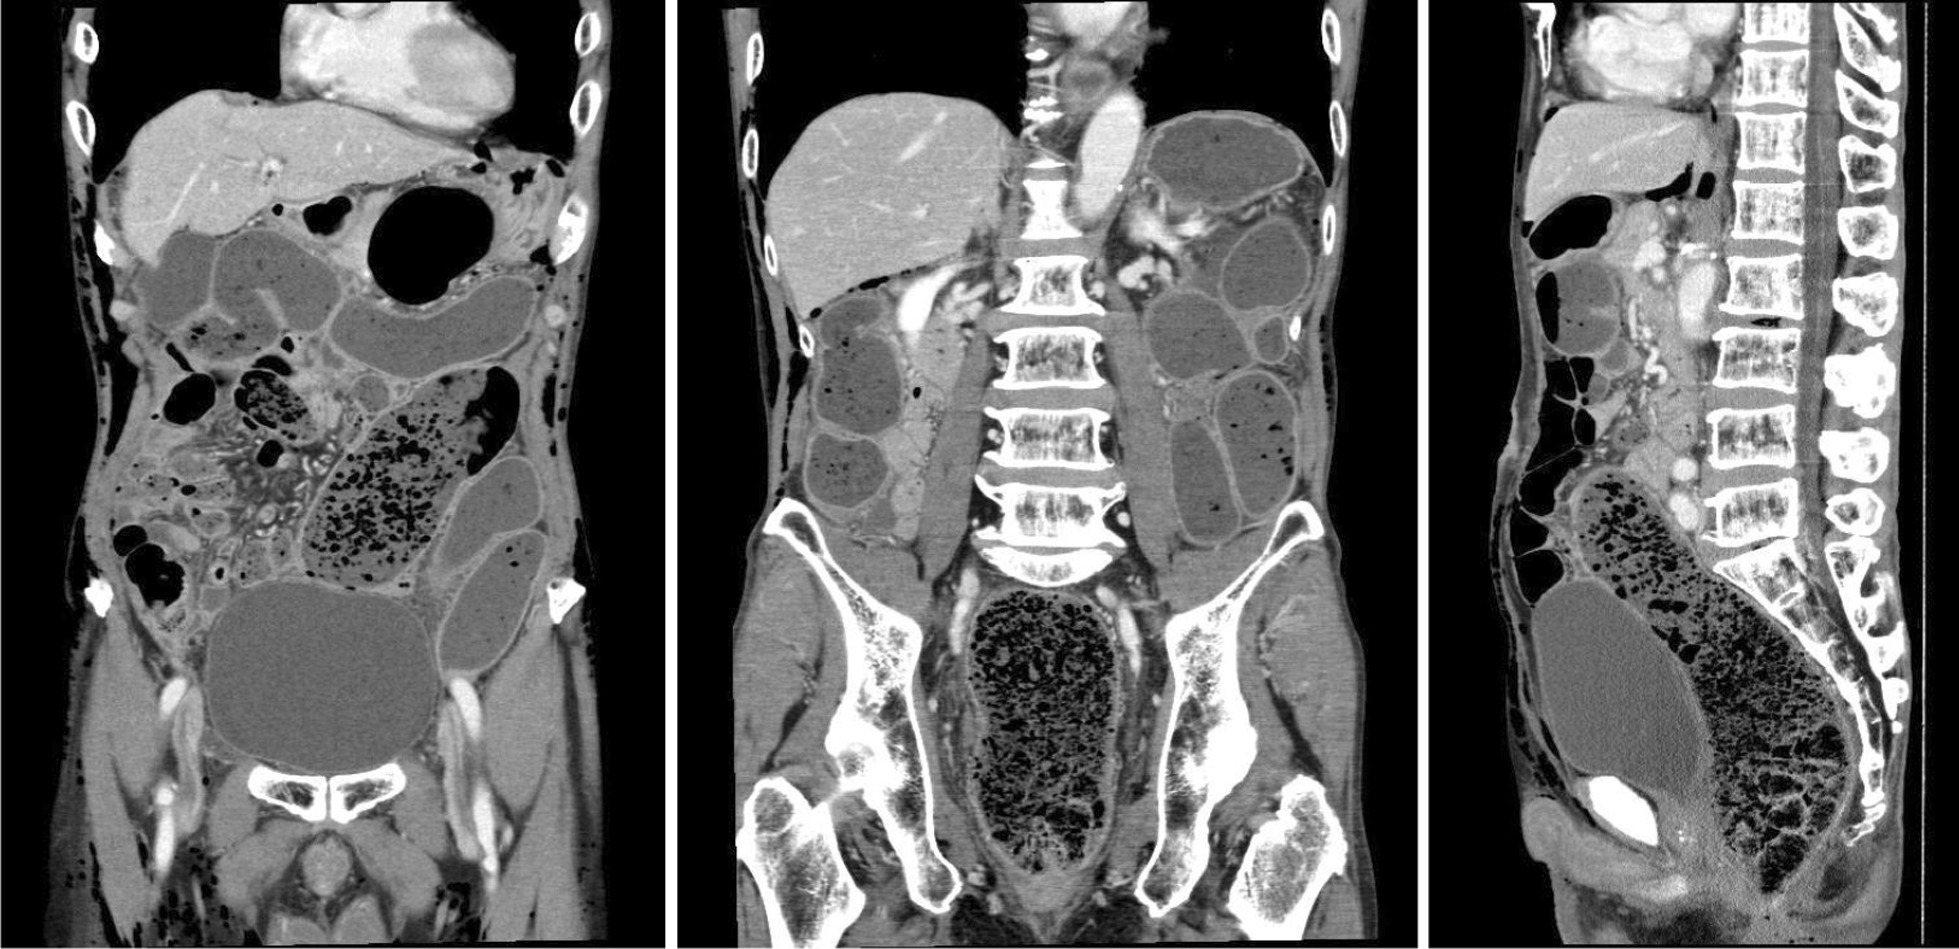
Enhanced computed tomography (CT) on admission revealed significant enlargement from the cecum to the rectum. However, small intestinal dilatation, mechanical obstruction, ischemia, and perforation were not observed
